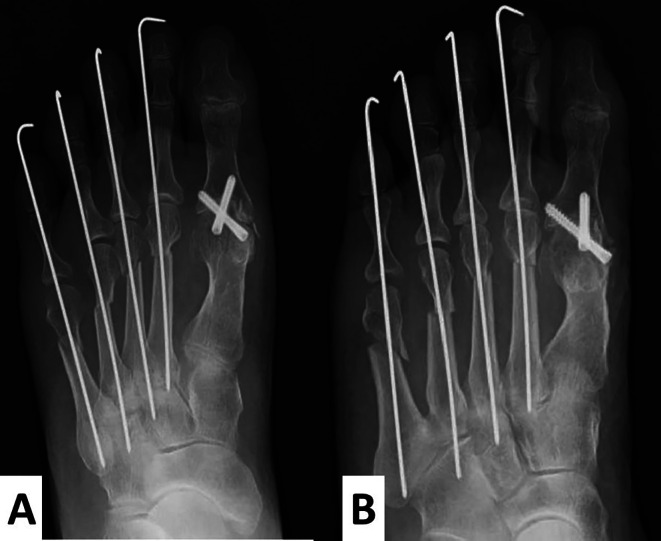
Radiographs after the operation. A cup and cone reamer are used to preserve the length of the hallux as much as possible. The hallux valgus angle is fixed at 0°, and the 1
